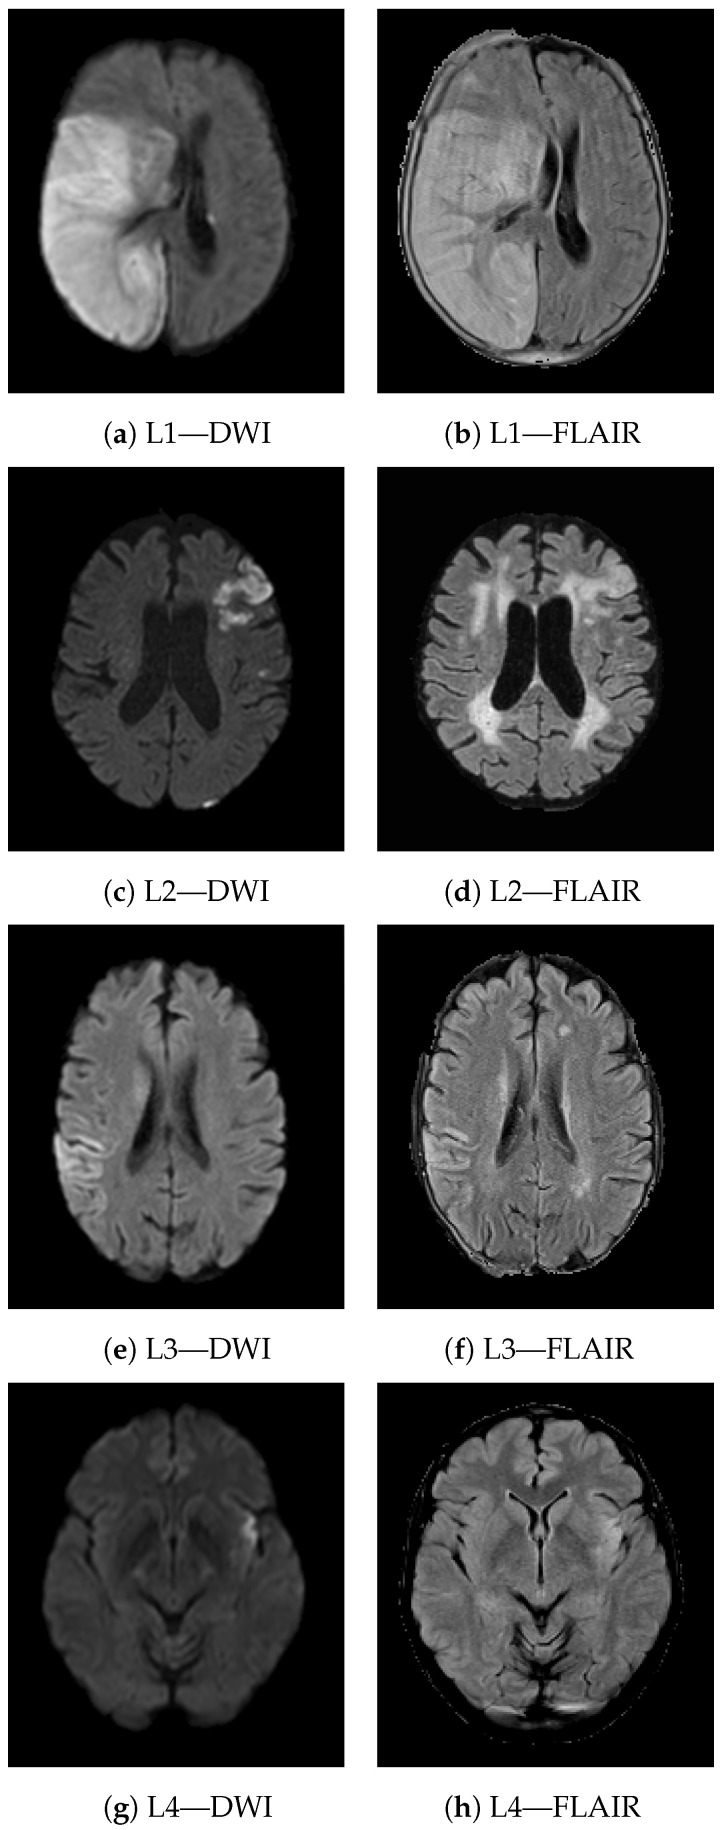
Axial views of DWI and T2-FLAIR scans from four different lesions (L1–L4). The lesions look hyperintense on both imaging sequences. L1 and L2 are two examples of patients with poor long-term functional outcome (90-day mRS of 6 and 5 respectively). L1 is quite extensive and presents PH1 hemorrage. Both lesions include WM and GM areas of the brain (texture heterogeneity measured in terms of Dependence non uniformity normalized—GLDM on DWI of 0.23 and 0.13, respectively). L3 and L4 instead are two examples of patients with good long-term functional outcome (90-day mRS of 2 and 0, respectively). Both lesions involve GM only (texture heterogeneity measured in terms of Dependence non uniformity normalized - GLDM on DWI of 0.08 and 0.04, respectively).
